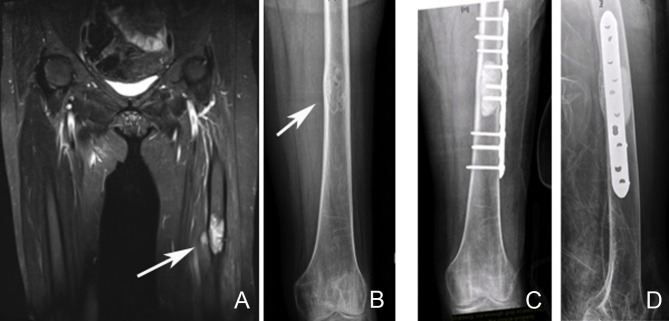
Left femoral diaphyseal metastatic lesion from breast carcinoma treated with curettage followed by cement-plate surgical fixation. (A) MRI showing a left femur diaphyseal intramedullary metastatic lesion. (B) The same lesion seen on plain X-ray. Note the mixed lytic sclerotic appearance of the lesion. (C,D) AP and lateral post-operative X-rays after curettage and cement-plate surgical fixation.
